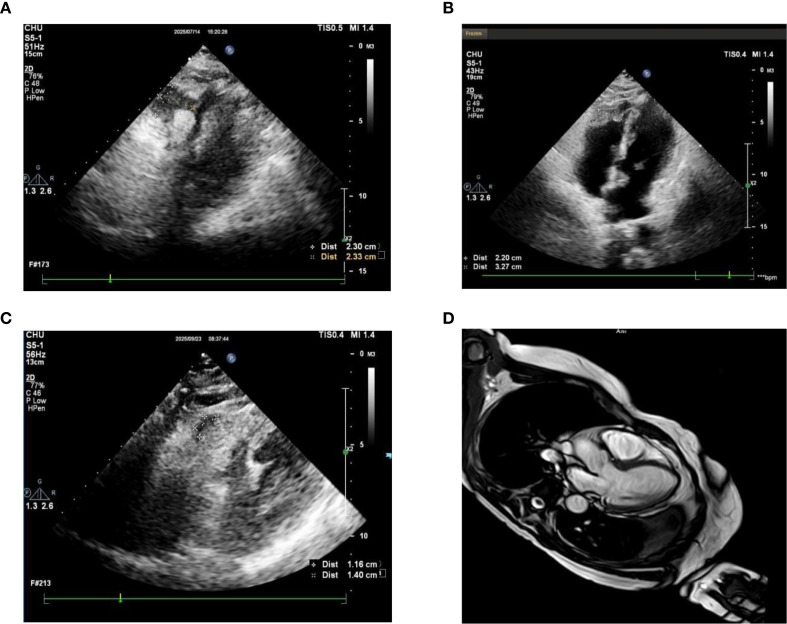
Follow-up cardiac imaging: transthoracic echocardiography and cardiac MRI demonstrating progressive shrinkage of the right ventricular mass. (a) Transthoracic echocardiography on 19 July 2025, showing the right ventricular mass. (b) Transthoracic echocardiography on 19 July 2025, showing the right ventricular mass, comparable to the July 14 image. (c) Transthoracic echocardiography on September 23, 2025, demonstrating marked reduction of the right ventricular mass. (d) Cardiac MRI showing the right ventricular mass on September 23, 2025.
